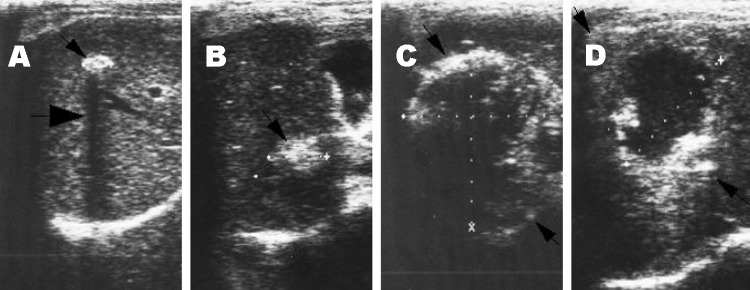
Lesions of alveolar echinococcosis (AE) by abdominal ultrasound examination. A) Calcified lesion: hyperechoic structure with a typical posterior shadow. B) Nodular hyperechoic lesion. C) Typical AE lesion: nonhomogeneous hyperechoic partially calcified area, without central necrosis. D) Typical AE lesion with central necrosis.
